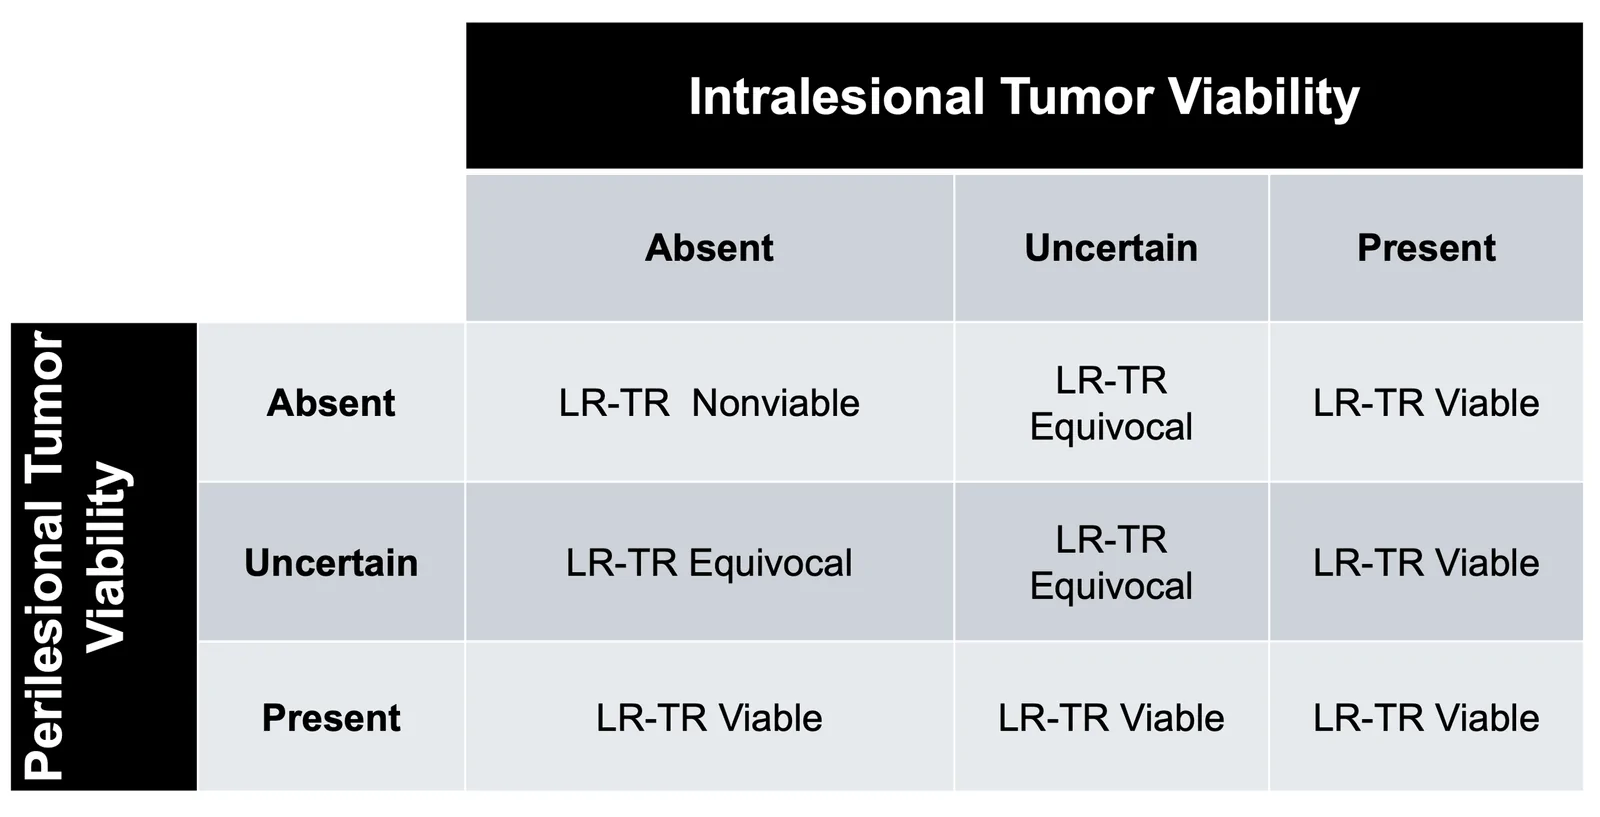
Decision matrix for CEUS LI-RADS Treatment Response Assessment (TRA) category assignment based on intralesional and perilesional tumor viability. Adapted and redrawn by the authors from the American College of Radiology CT/MRI LI-RADS Treatment Response Algorithm v2024 [15].
